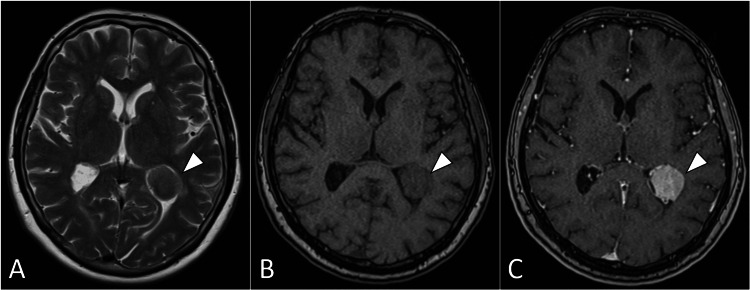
Axial brain MRI images featuring T2-weighted image (A), T1-weighted image (B), and post-contrast image (C). The images reveal a distinct intraventricular lesion (arrowhead) situated in the occipital horn of the left lateral ventricle, exhibiting pronounced solid contrast enhancement—a characteristic indicative of meningioma. MRI: magnetic resonance imaging
